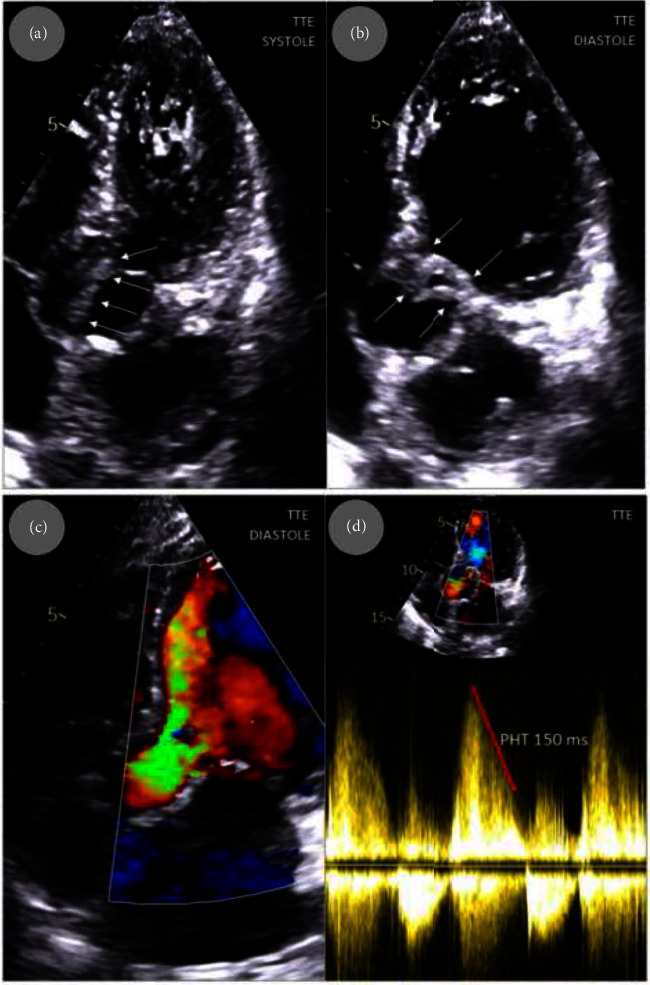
Echocardiography at admission: large, longitudinal, highly mobile vegetation (white arrows) on aortic valve moving in systole into aortic root (a) and presenting as rolled up structure in diastole (b); massive aortic regurgitation in color Doppler imaging (c); and in spectral Doppler imaging—see very short pressure half time (red arrow) (d). TTE, transthoracic echocardiography, PHT, pressure half time.
